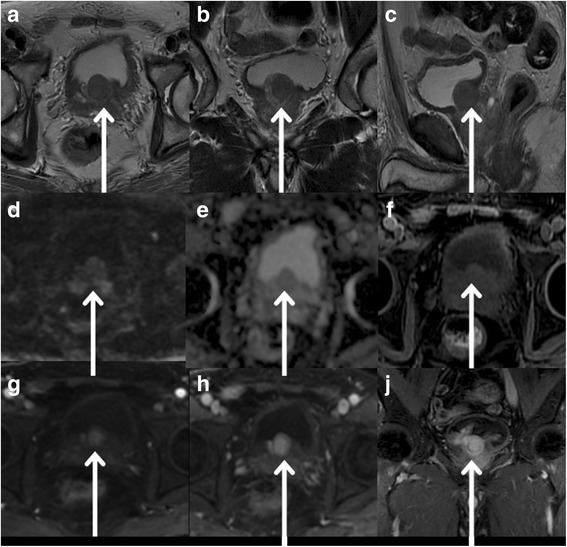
MR images of prostatic leiomyoma. a, b, c Axial, Coronal, and Sagittal High-resolution T2WI images show a well-circumscribed nodule with a capsule (homogeneous low signal intensity) in the bottom of prostatic left inner zone as homogeneous and slightly hypointense signal compared to the muscle (arrows). d On ultra-high b-value DWI (b = 3000 s/mm^2^), slightly high signal nodule compared to the muscle (arrows) was observed. e On ultra-high b-value DWI apparent diffusion coefficient map, ADC value was 0.817±0.016 × 10^−3^ mm^2^/s. f On T1WI MRI with fat suppression (Pre scanned imaging), the homogeneous isointense signal of nodule compared to the muscle was found. g, h, j On Axial contrast T1WI during the arterial phase, the tumor was strongly and homogeneously enhanced. During venous and delay phases, the tumor showed continuous signal increase (arrows). The margin of tumor was clear, and the envelope was not enhanced
